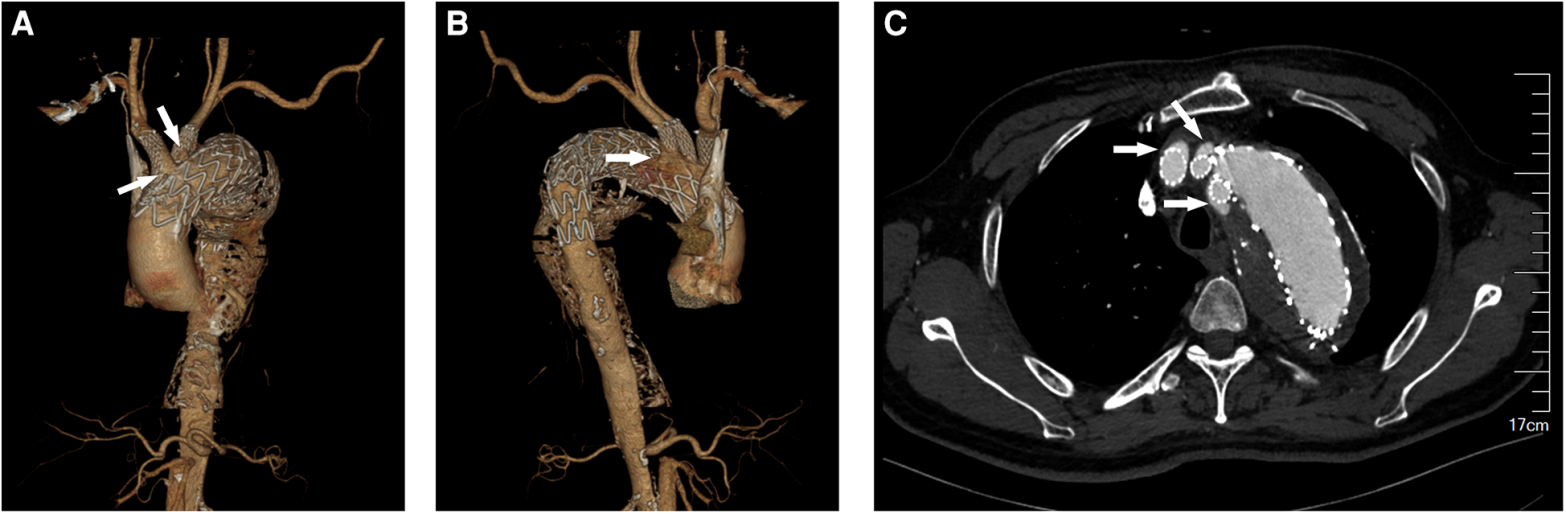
A 69-year-old male patient underwent fb-arch repair for a postdissection aortic arch aneurysm. Probably because of the large aortic arch diameter, there seems to be endoleaks around the reconstructed supra-aortic vessels during the follow-up. (A) The CTA imaging of the Type IIIc endoleaks near the brachiocephalic trunk and the left common carotid artery after fb-arch repair. (arrow). (B) The CTA imaging of the Type IIIc endoleak near the left subclavian artery after fb-arch repair. (arrow). (C) The cross-sectional image of the Type IIIc endoleaks (arrow).
